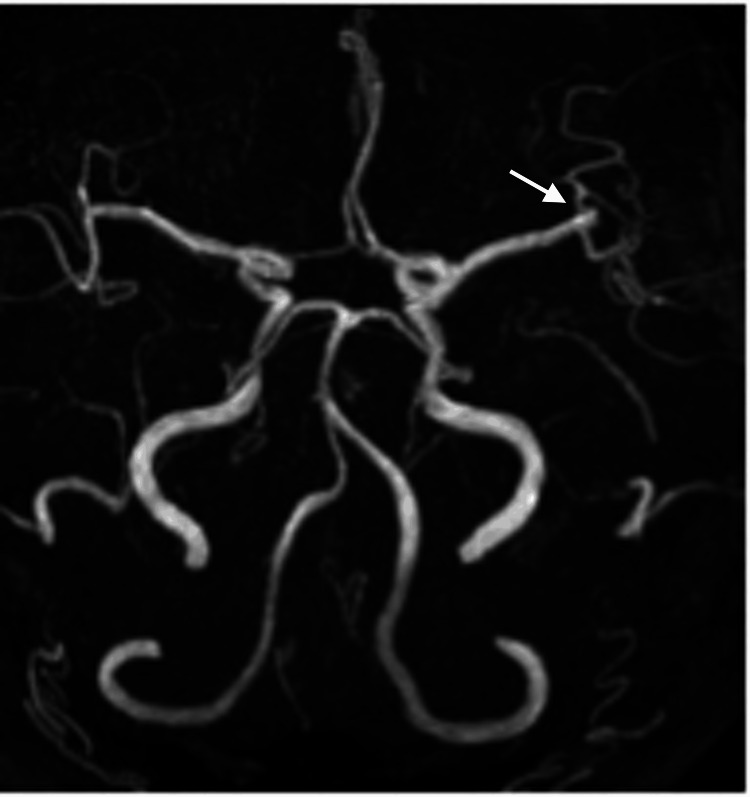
Preoperative magnetic resonance imaging A 3.8 mm-diameter unruptured saccular aneurysm (white arrow) at the bifurcation of the left middle cerebral artery (MCA).
